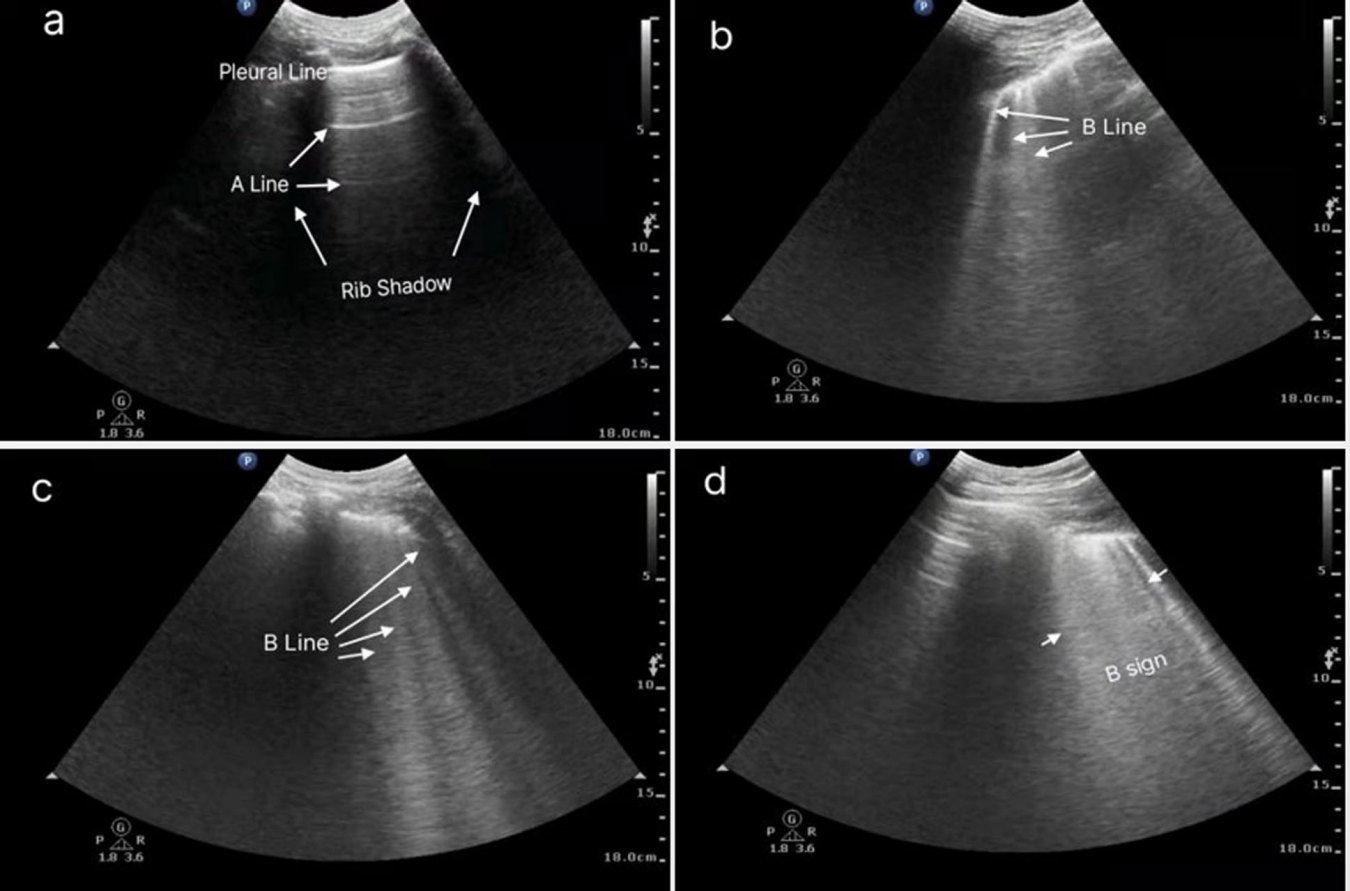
Example of lung ultrasound B-line score. a, b, c, and d correspond to scores of 0, 3, 4, and 5, respectively
